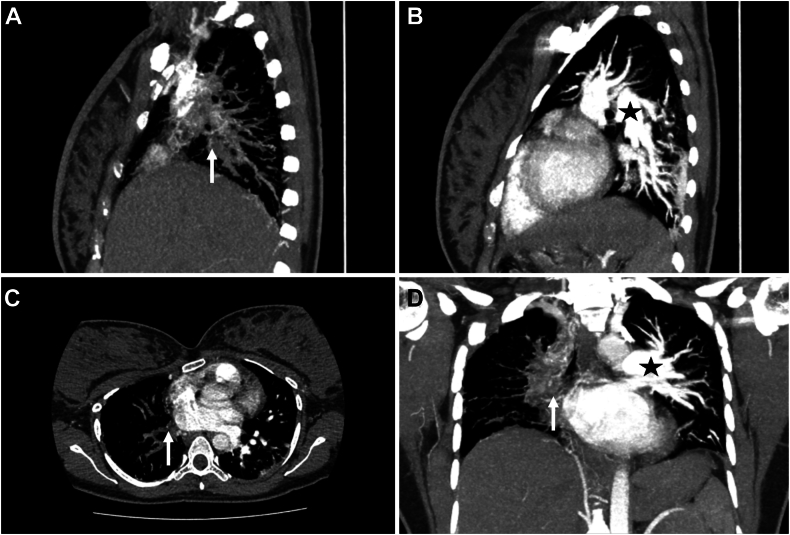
Chest CT with contrast demonstrates a filling defect seen where an RPA (*white arrows*) should arise from the main pulmonary artery trunk in the (A) right lateral sagittal, (B) left lateral sagittal, (C) axial, and (D) coronal planes. For comparison, the left pulmonary artery (*black stars*) can be seen.
